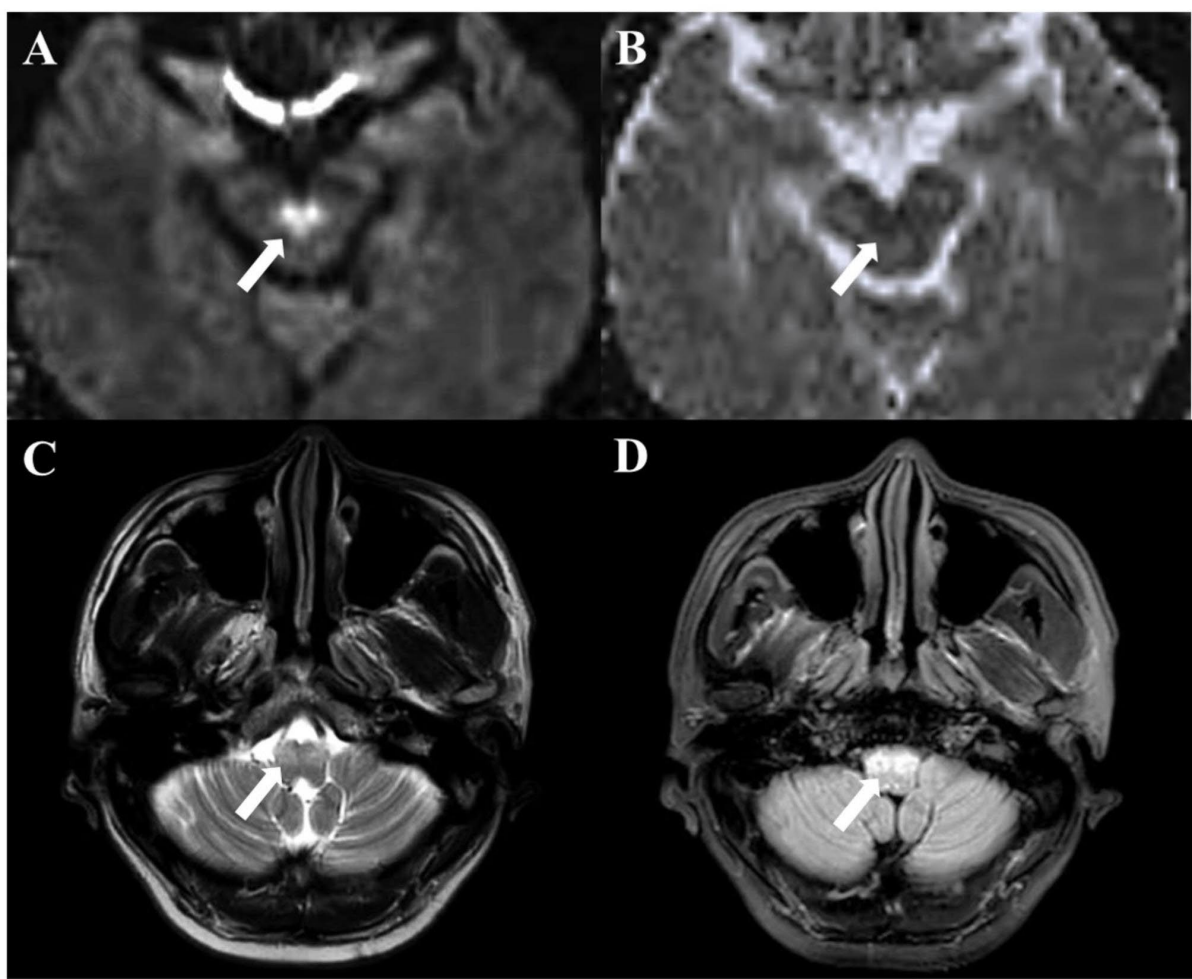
Brain magnetic resonance imaging axial sections. (A) Diffusion weighted imaging and (B) apparent diffusion coefficient sequences showed diffusion restriction in the caudal midbrain region 3 years prior to this admission. (C) T2-weighed and (D) fluid-attenuated inversion recovery images showed bilateral swollen and hyperintense olives during this admission
